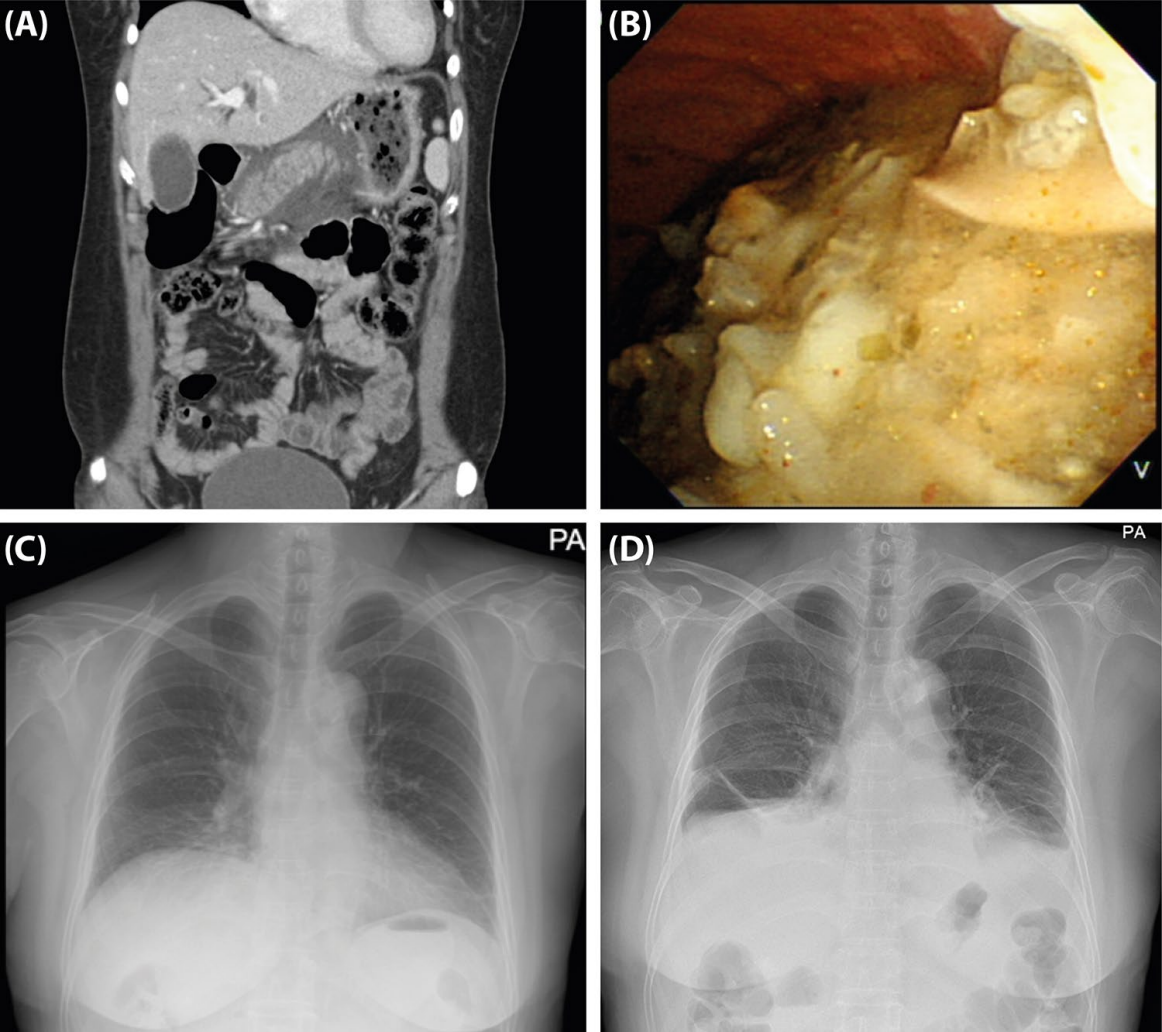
Imaging of patients who underwent ERCP within 18 h of symptom onset. (A) Emergency room computed tomography (CT) scan demonstrating peripancreatic fluid collection and edema around the pancreas, as well as residual food in the stomach. (B) Large amount of residual food in the stomach during ERCP. (C) Chest X-ray taken before ERCP. (D) Chest X-ray taken after ERCP, demonstrating pleural effusion in both lower lungs and aspiration pneumonia lesions in the left lower lobe.
